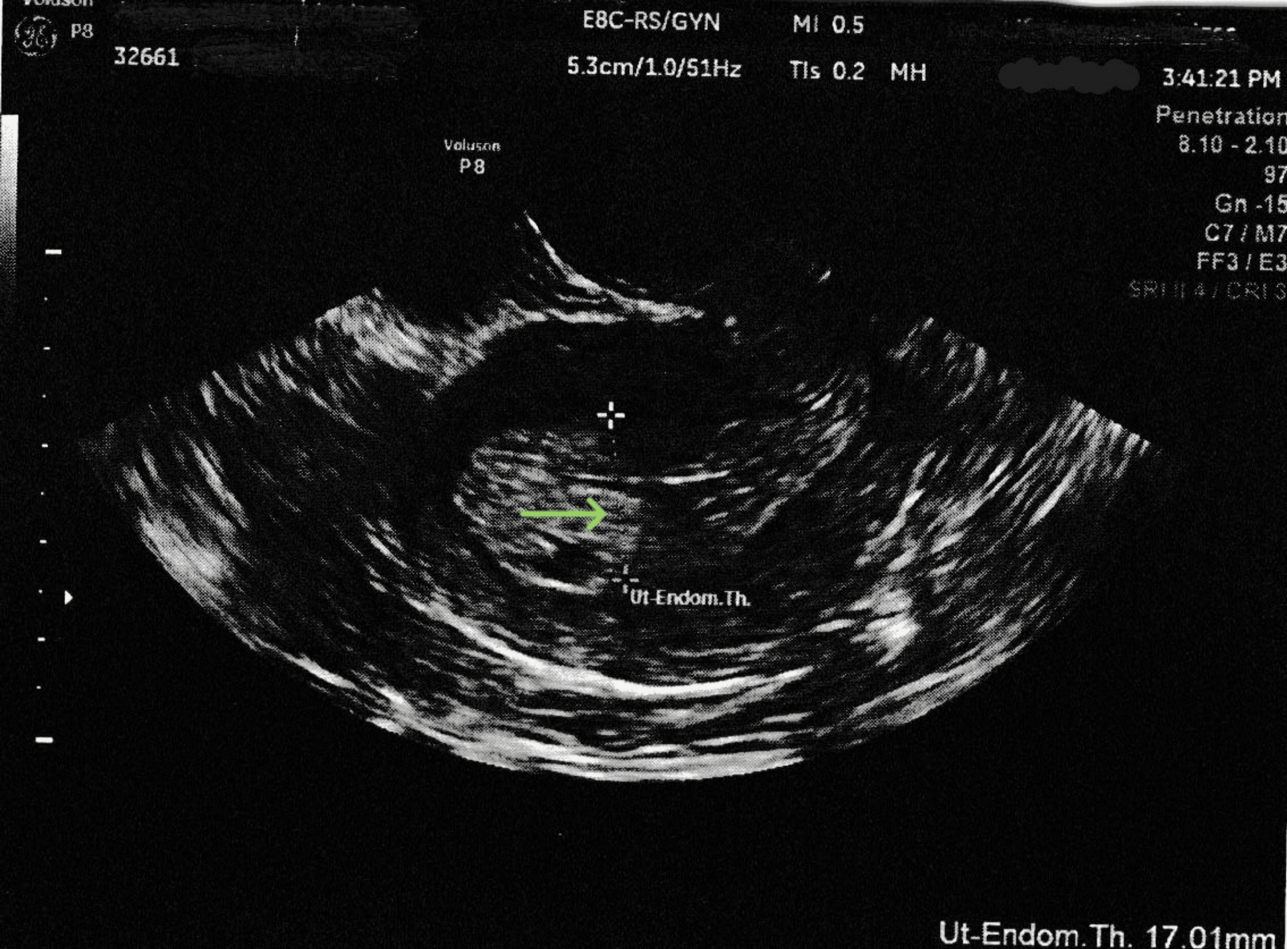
Pelvic transvaginal ultrasound showing an endometrial thickness of 17 mm
